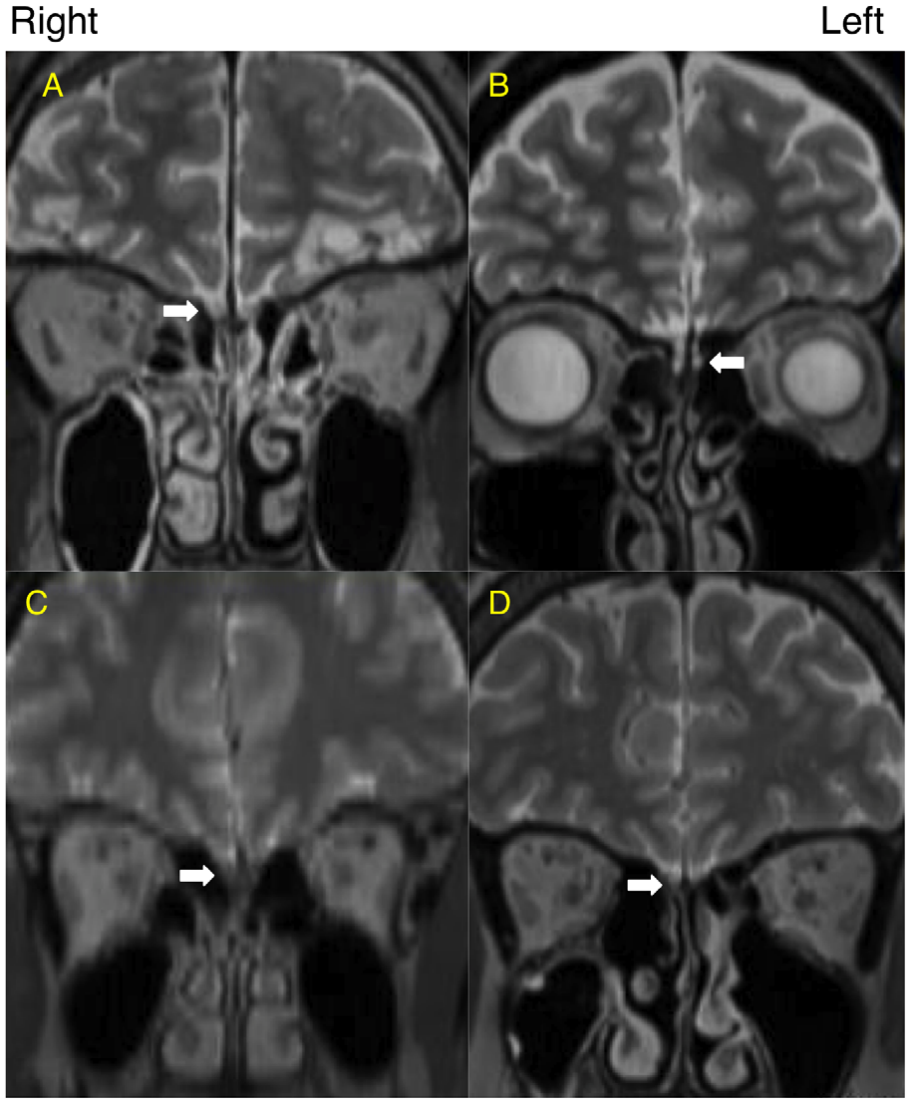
MRI image (T2 weighted, coronal view). White arrows indicate the olfactory bulb and olfactory nerve. (A) A 60-year-old healthy male volunteer. (B) A 44-year-old female with hyposmia after head trauma. (C) A 42-year-old female with hyposmia after upper respiratory tract infection. (D) A 67-year-old female with hyposmia due to chronic rhinosinusitis.
